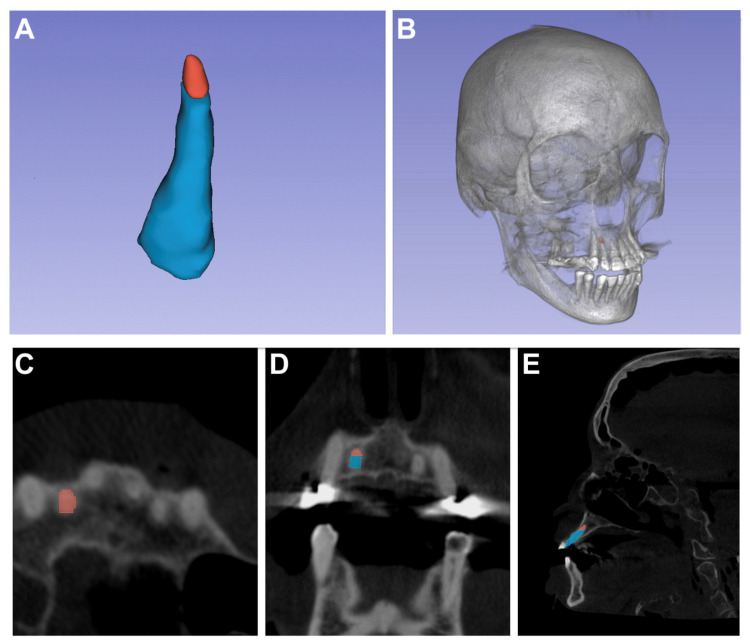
Preoperative rendering of the right maxillary lateral incisor using 3D-Slicer^®^ software. (A): A three-dimensional model of the tooth, with the apical 3 mm of the root tip individually color-coded (red). (B): A model of the skull with highlighted red root tip (3 mm of the apical root tip of the lateral right incisor). (C): An axial plane of the cone beam computed tomography (CBCT) scan presenting the apical root tip in red. (D): A frontal view of the CBCT scan displaying both the root tip (red) and the root (blue). (E): The left maxillary lateral incisor marked in a sagittal CBCT plane.
